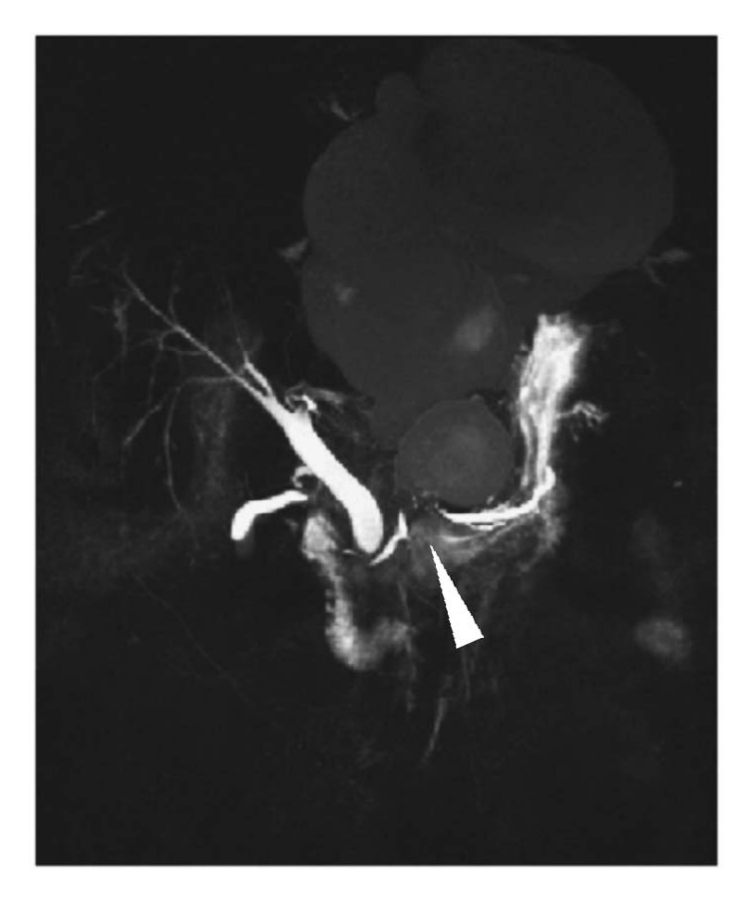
T2-weighted coronal magnetic resonance imaging of the upper abdomen and magnetic resonance cholangiopancreatography. There is communication between mediastinal and abdominal pseudocysts through the esophageal hiatus. High-grade ductal stenosis (arrowhead) is shown, but only a slight widening in the pancreatic body and tail.
